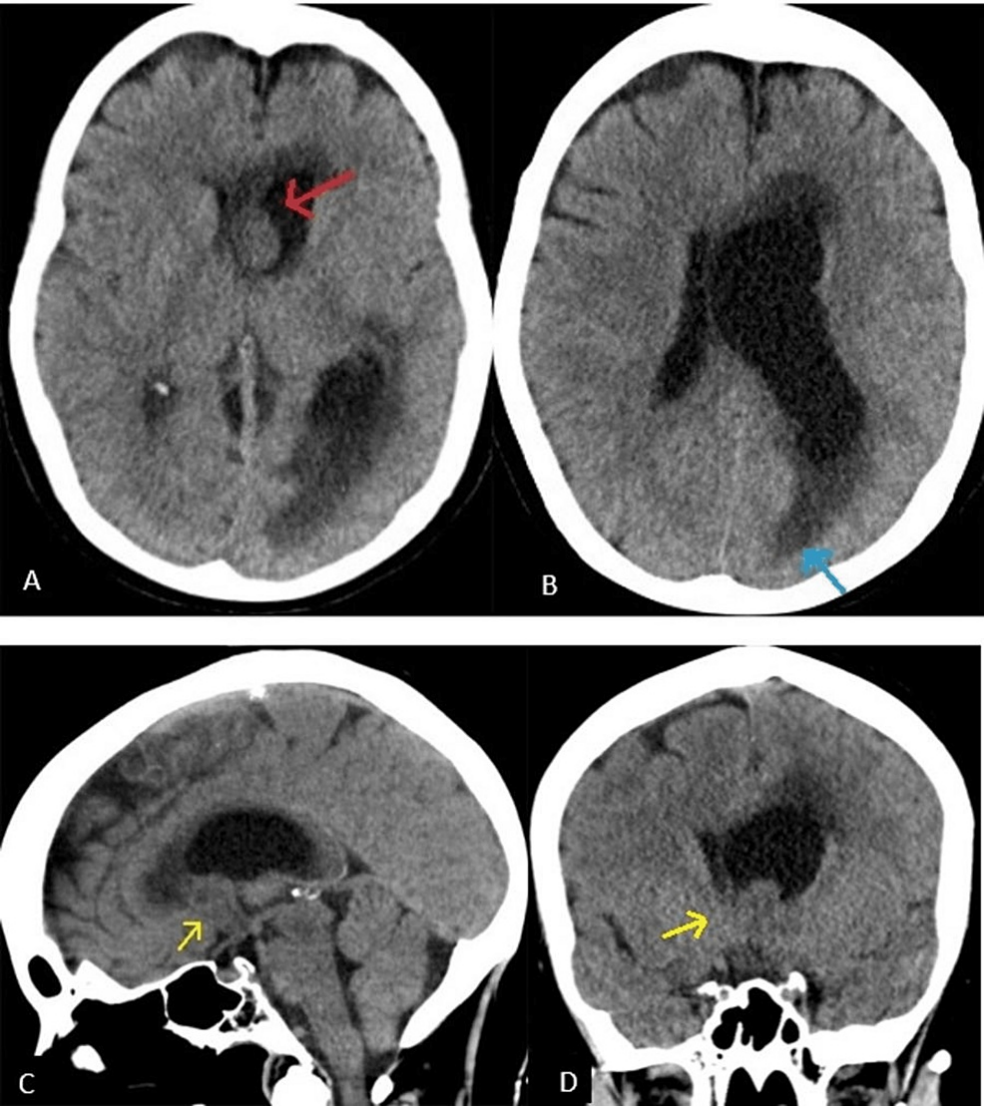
CT findings Axial (a and b), sagittal (c), and coronal (d) non-enhanced CT images of the brain. An intraventricular nodule is demonstrated (red arrow) with asymmetric dilation of the left lateral ventricle. There is a midline shift, most notable at the level of the interventricular septum. Periventricular hypoattenuation (blue arrow) is suggestive of transependymal edema, an effect of acute hydrocephalus. There is an extension of the nodule into the foramen of Monro (yellow arrow).
